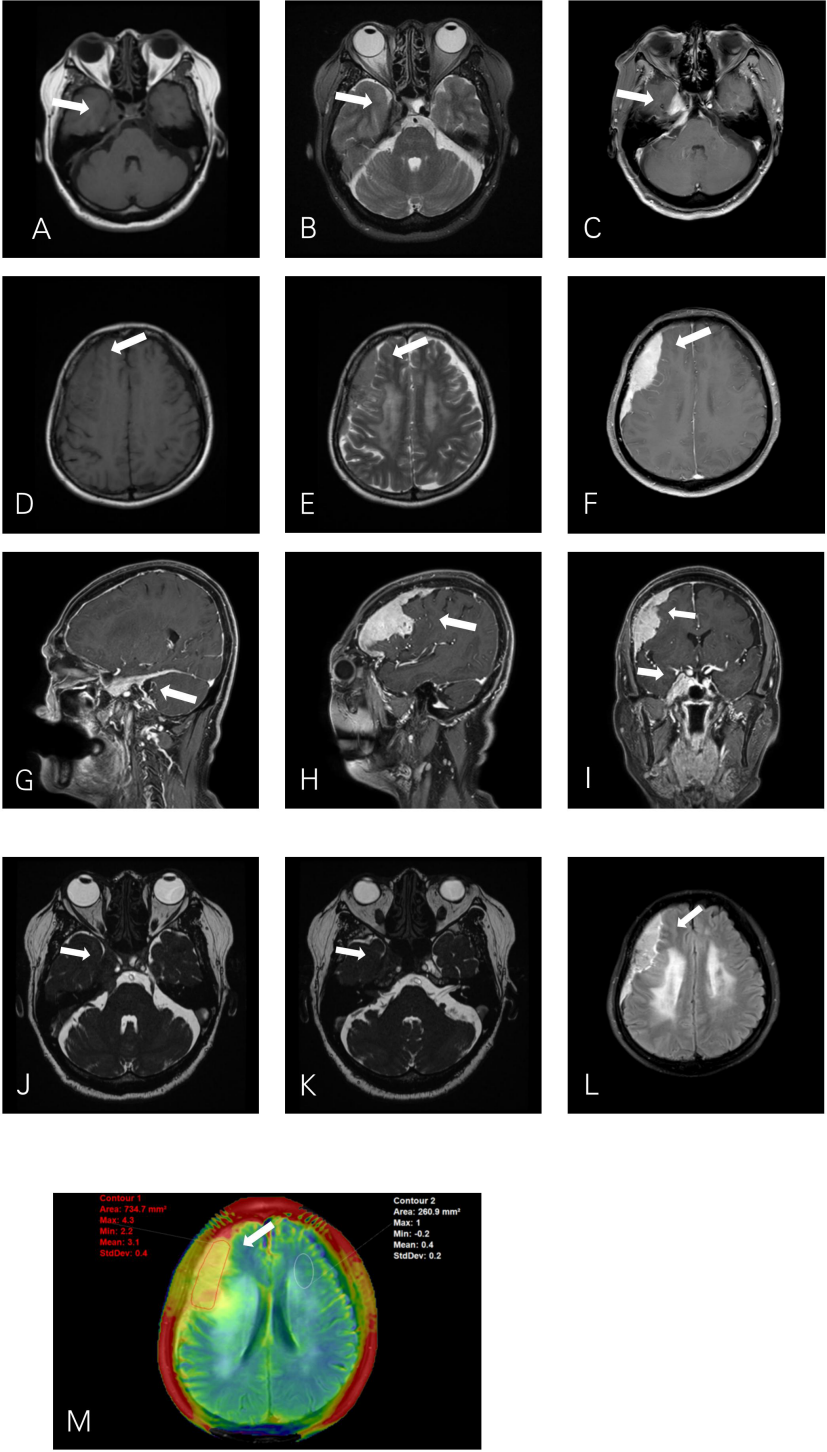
Axial T1WI (A) and T2WI (B) show a fusiform lesion isointensity to gray matter in the right cavernous sinus (CS). T1WI (D) and T2WI (E) show a crescent–shaped extra–axial mass in the right fronto–temporo–parietal region dura. The right CS lesion (J) extends into the posterior cranial fossa into the internal auditory canal and encircles the auditory nerve and facial nerve (K). Post–contrast T1–weighted (C, F, G, H, I) images show intense homogeneous enhancement of the mass lesions with both dural tails visible. The leptomeningeal involvement of the right extra–axial dura lesion shows serrated enhancement on contrast–enhanced T2–FLAIR (L). APTw image (M) shows a relative homogeneous tumor mass, with APTw signal intensity rates of 2.2%–4.3% (mean: 3.1%).
